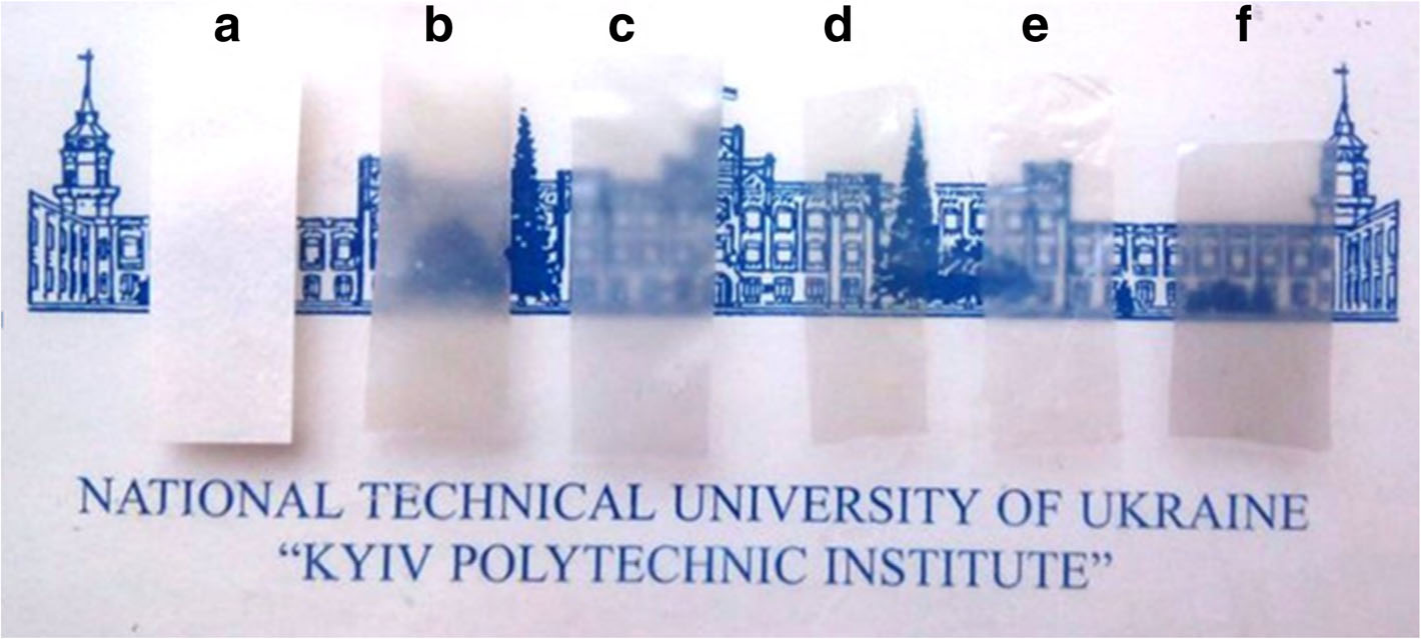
Transparent sheets prepared from cellulose dispersion after grinding (a), after grinding and hydrolysis by sulfuric acid of concentration 18 % (b), 30 % (c), 43 % (d), 50 % (e), and 64 % (f) with ultrasound treatment
